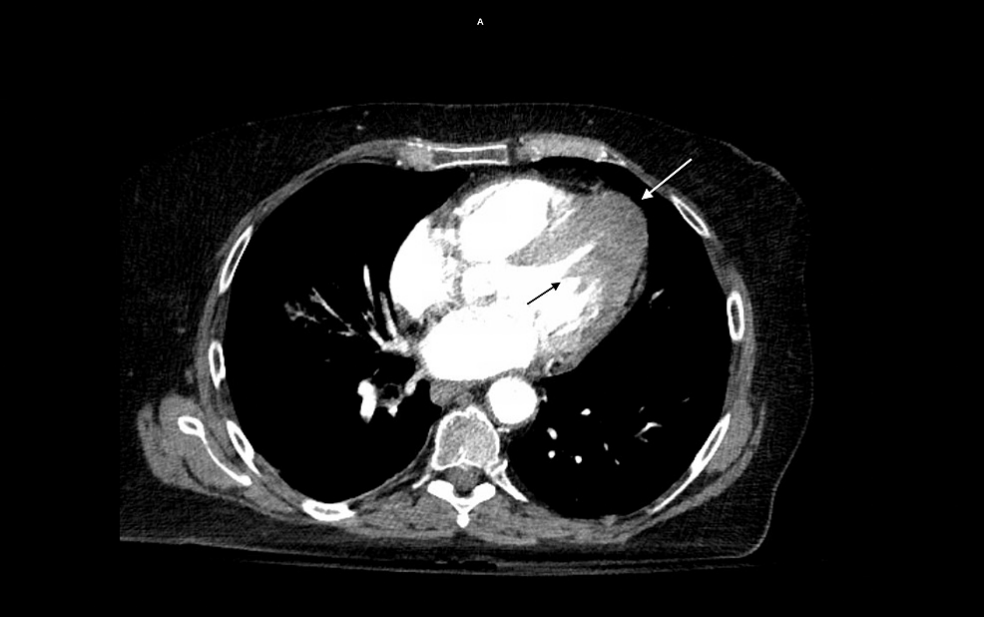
CT with contrast demonstrating apical hypertrophy and ace-of-spades appearance of the left ventricle
